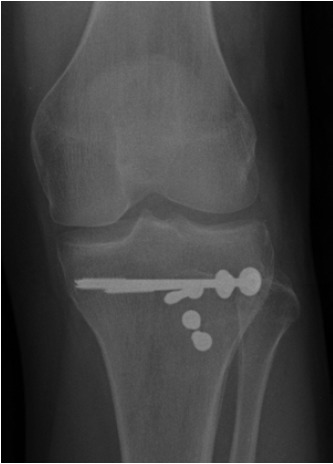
Post-operative anteroposterior radiograph of the left
